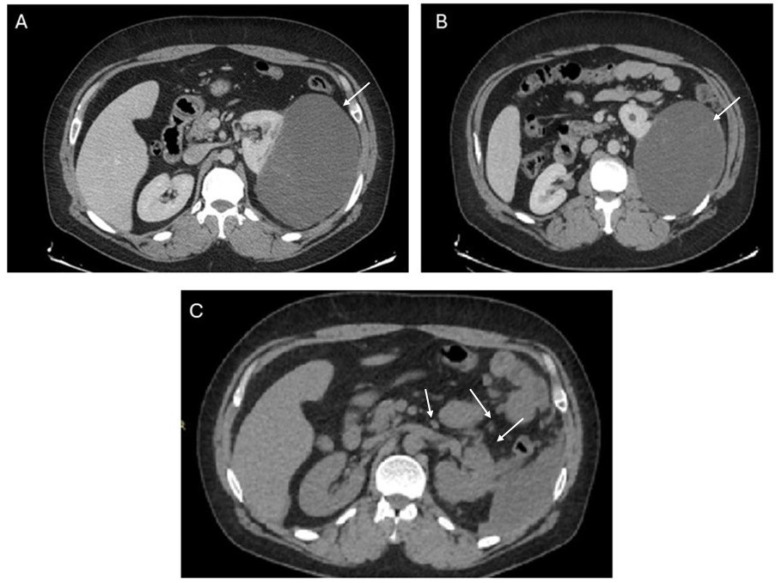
Imaging findings. (A,B) Pre-operative CT scans: exophytic, homogenous mass on the left kidney (arrow); (C) post-operative CT scan showing the presence of solid abdominal and peri-renal implants (arrows).
